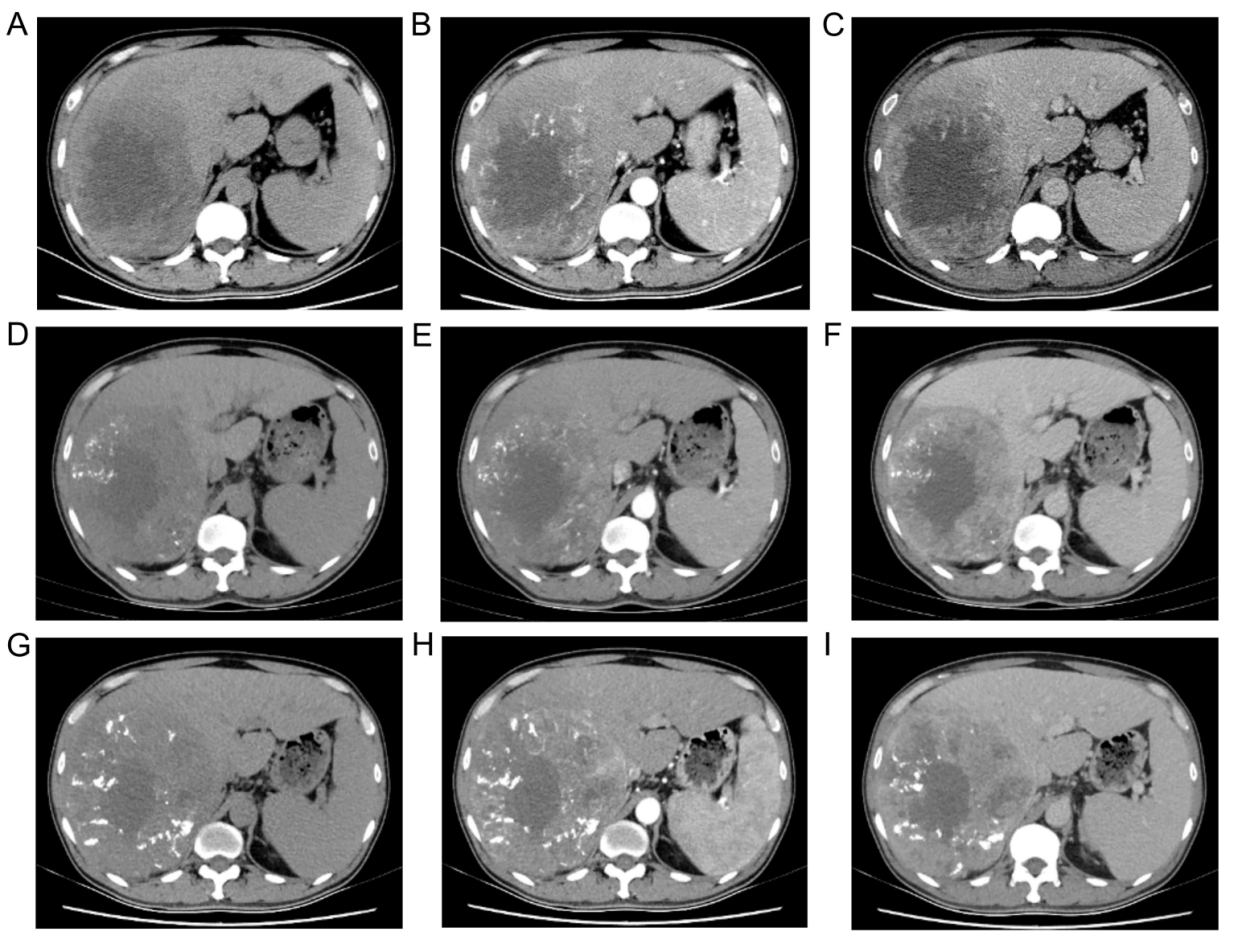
A 42-year-old male with alcohol-associated cirrhosis history. The pre- transarterial chemoembolization (TACE) dynamic CT shows a 130-mm tumor in the right hepatic lobe with obvious increase in arterial phase and decreased in portal phase (A-C). The dynamic enhanced CT after the initial TACE (D-F) and the second TACE (G-I) show a viable tumor >50%.
